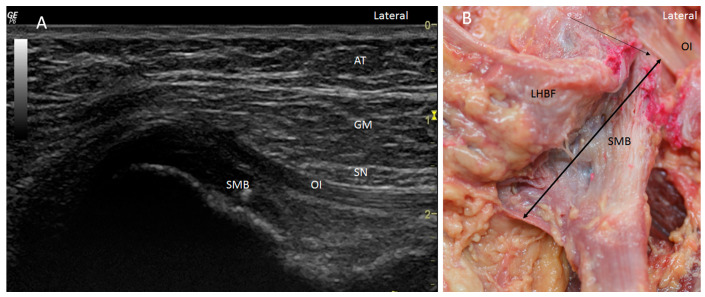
(A) Ultrasound study in the short axis on the right side showing the high origin semimembranosus muscle (SMB). It goes up even as far as to be placed under the obturator internus muscle (OI). SN: sciatic nerve GM: gluteus maximus muscle; AT: adipose tissue. (B) Anatomical view of the long and high origin of the tendon of the SMB (black arrow) under the OI after moving the LHBF medially on the right side.
